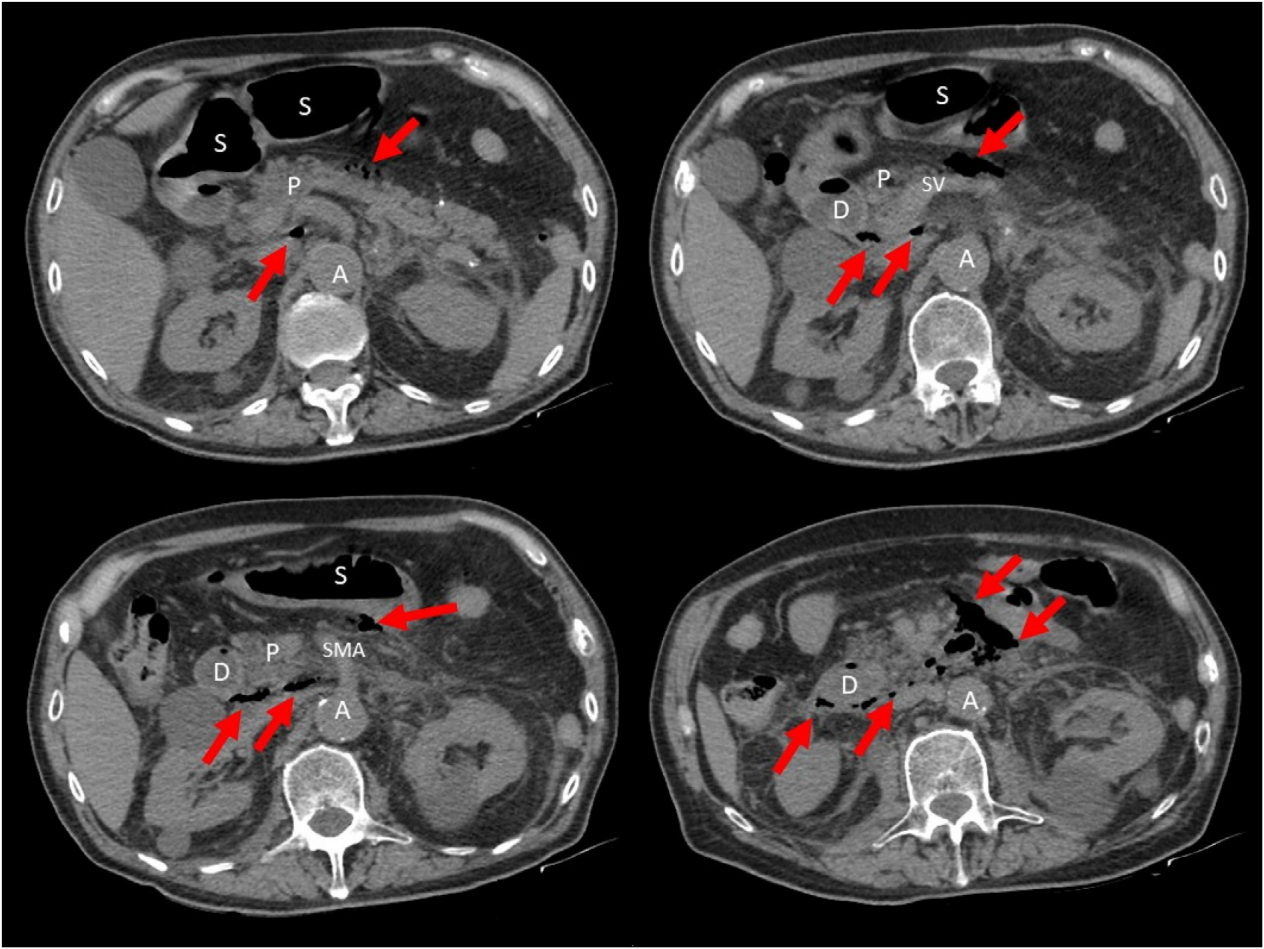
Computed tomography of the abdomen showing retroperitoneal fluid with free air (red arrows), suggestive of perforation. (P: pancreas; A: aorta; S: stomach; D: duodenum; SV: splenic vein; SMA: superior mesenteric artery). (For interpretation of the references to colour in this figure legend, the reader is referred to the Web version of this article.)
